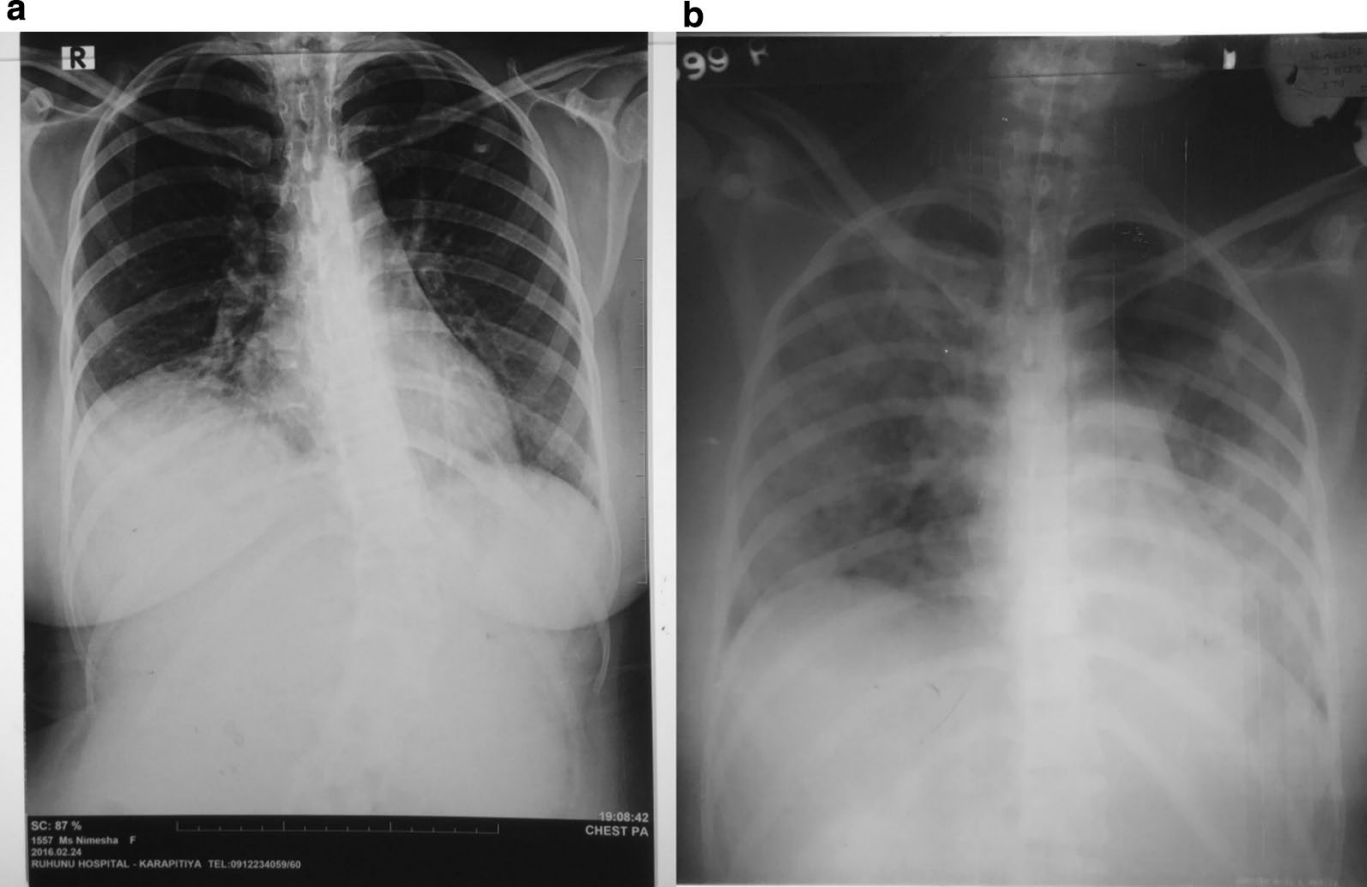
a Chest radiograph on admission. Shows slight obliteration of medial half of diaphragmatic border suggestive of right lower lobe consolidation. b Chest radiograph on day 3. Shows multi lobar consolidation
